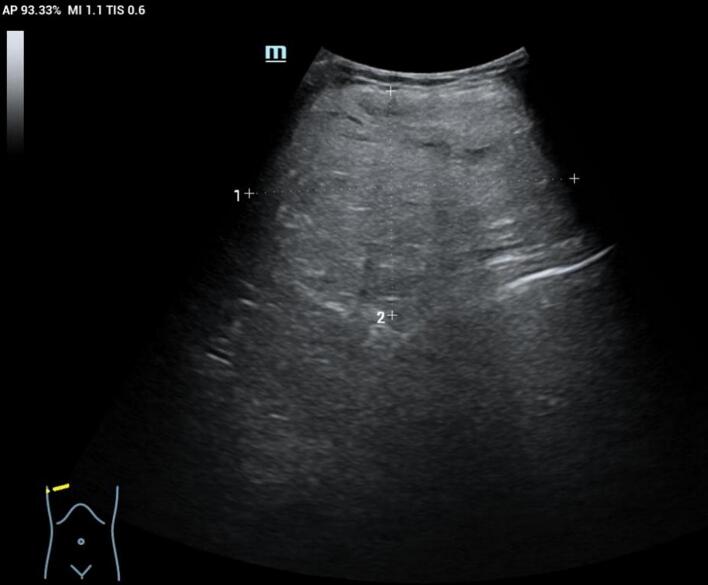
Breast ultrasound shows heterogeneous and slightly strong echoes in the upper quadrant of the right breast, with a regular shape and visible boundaries.
